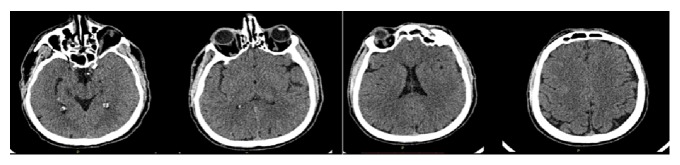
Normal finding postoperative brain CT.
